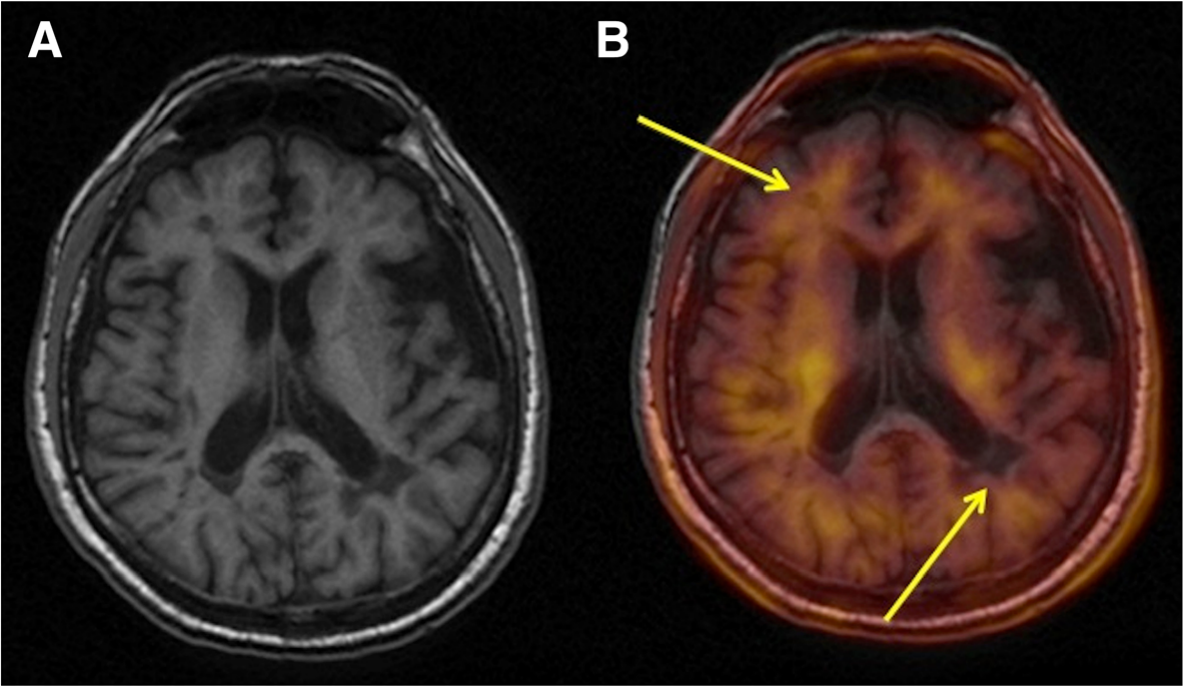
Co-registered MRI and ^18^F-florbetaben-PET images. a T1-weighted MRI. b Co-registered MRI and ^18^F-florbetaben-PET image. Arrows show lesions and areas of low uptake detected using PET
